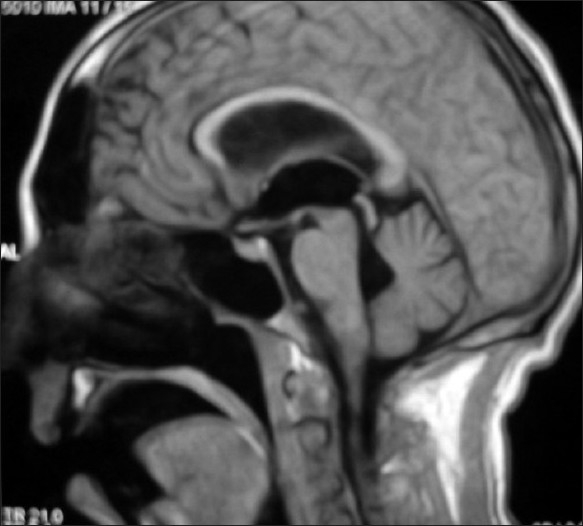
T1W sagittal MRI showing no obvious obstruction
